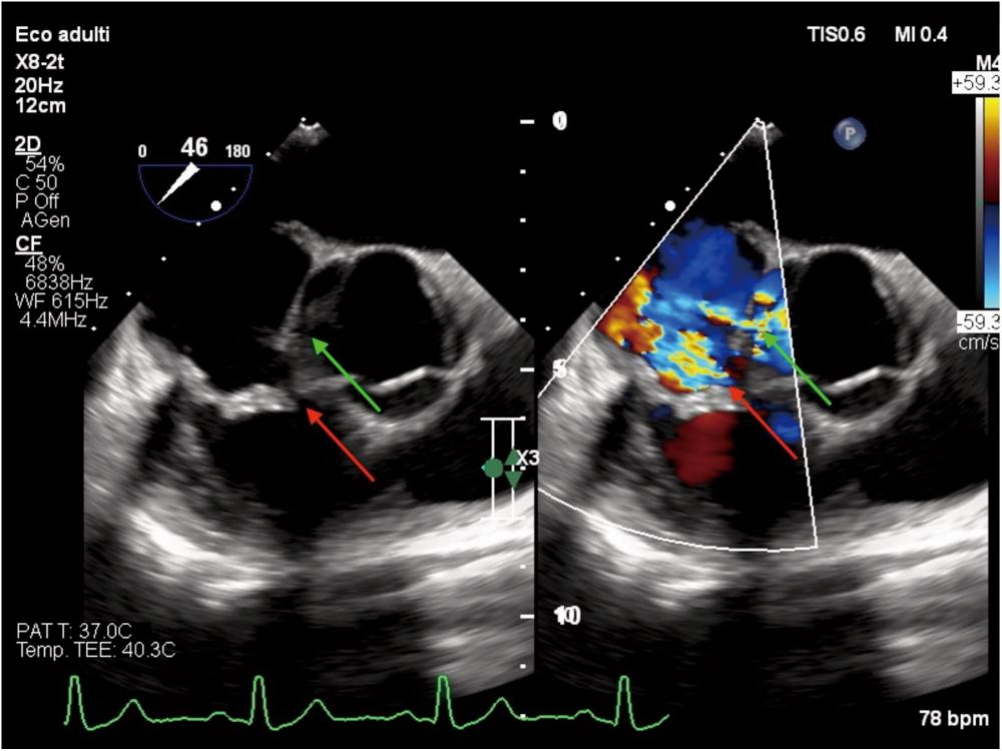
TEE (short axis), tricuspid regurgitation (red arrow) and aorta-right atrium’s shunt (green arrow) studied with colour Doppler. Two months after discharge, we can see result comparable to the post-operative examination.
